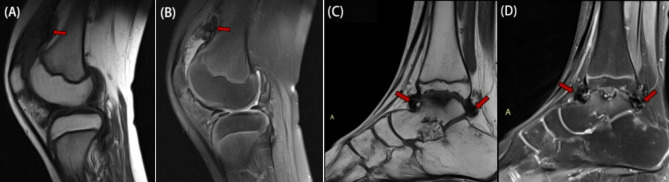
MRI Findings in Two Cases of Haemophilic Arthropathy. Figures 3A-B: An 11-year-old male with a history of haemophilia A since childhood, presenting with recurrent bleeding, swelling, and pain in the left knee. Sagittal T1-weighted imaging (T1WI) (A) and proton density-weighted imaging with fat saturation (PD-FS) (B) of the left knee joint. Figures 3C-D: A 10-year-old male with a similar history of haemophilia A since childhood, presenting with recurrent bleeding, swelling, and pain in the right ankle. Sagittal T1WI (C) and PD-FS (D) images of the right ankle joint are displayed. Haemosiderin appears as areas of extremely low signal intensity on both T1WI and PD-FS images. Haemosiderin deposition is closely associated with synovial hypertrophy on MRI, primarily located in the joint capsule folds and hypertrophic synovial tissue (red arrows)
